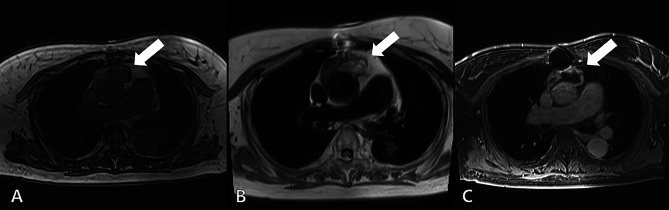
Preoperative chest MRI. (a) T1-weighted image suggestive of hematoma (white arrow). (b) HASTE T2-weighted sequence images suggesting the possibility of a pseudoaneurysm (white arrow). (c) T1 contrast image with rim enhancement (white arrow) MRI, magnetic resonance imaging; HASTE, half-Fourier single-shot turbo spin-echo
